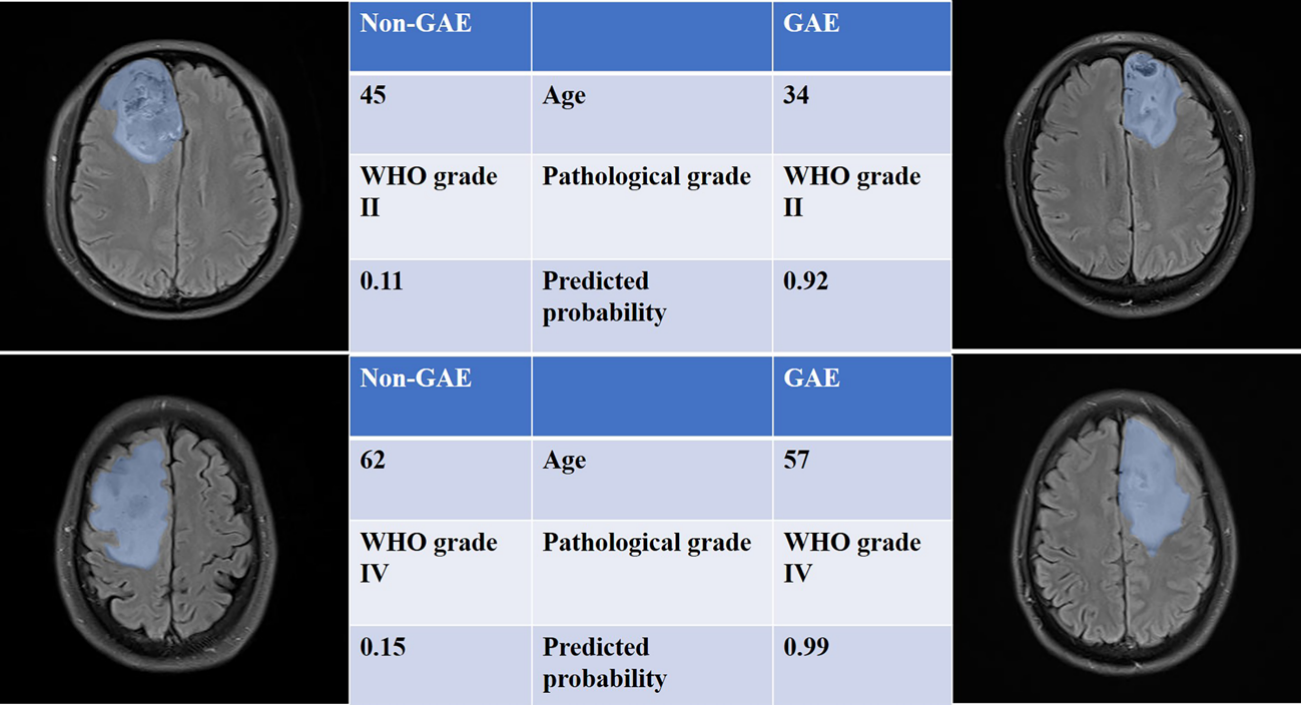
T2 FLAIR images of four patients with or without GAE experience. The blue shadow in the images was manually delineated as the region of VOI. All the clinical characteristics and predicted probabilities of the combined model are presented at the center of the table.
